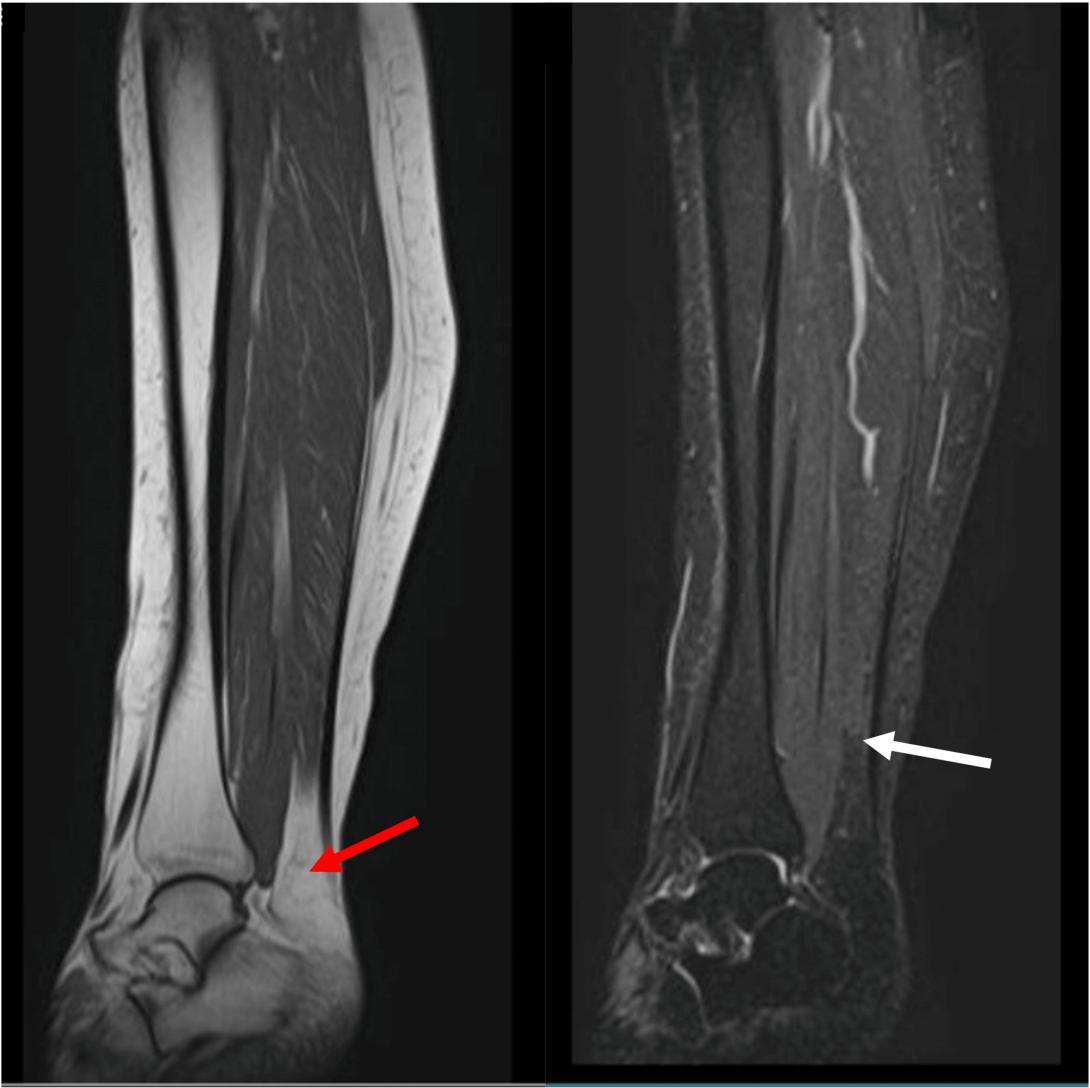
T1- and T2-weighted MRI of the left tibia Sagittal view T1 (left) and T2 (right) weighted MRI of the left tibia. The Achilles tendon (red arrow) appears intact on T1-weighted images, and there are no hyperintensities on T2-weighted images indicative of an inflammatory response within the myotendinous junction (white arrow).
